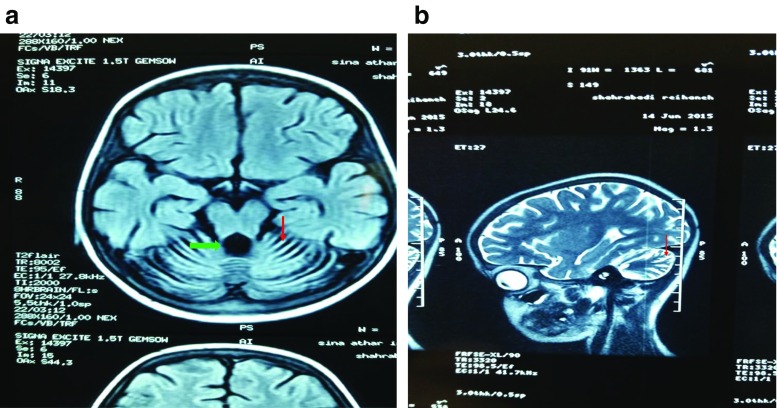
Brain MRI. Axial T1-weighted (a) and sagittal T2-weighted (b) brain MRI. The diffuse cerebellar volume loss with enlargement of cerebellar sulci (*red arrow*), vermian atrophy and compensatory enlargement of the fourth ventricle (*green arrow*)
